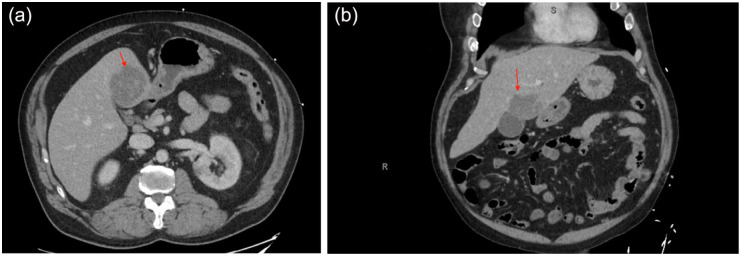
CTAP with contrast. (a) Axial view of hepatic abscess (red arrow). (b) Coronal view of hepatic abscess (red arrow). CTAP: computed tomography of the abdomen and pelvis.
